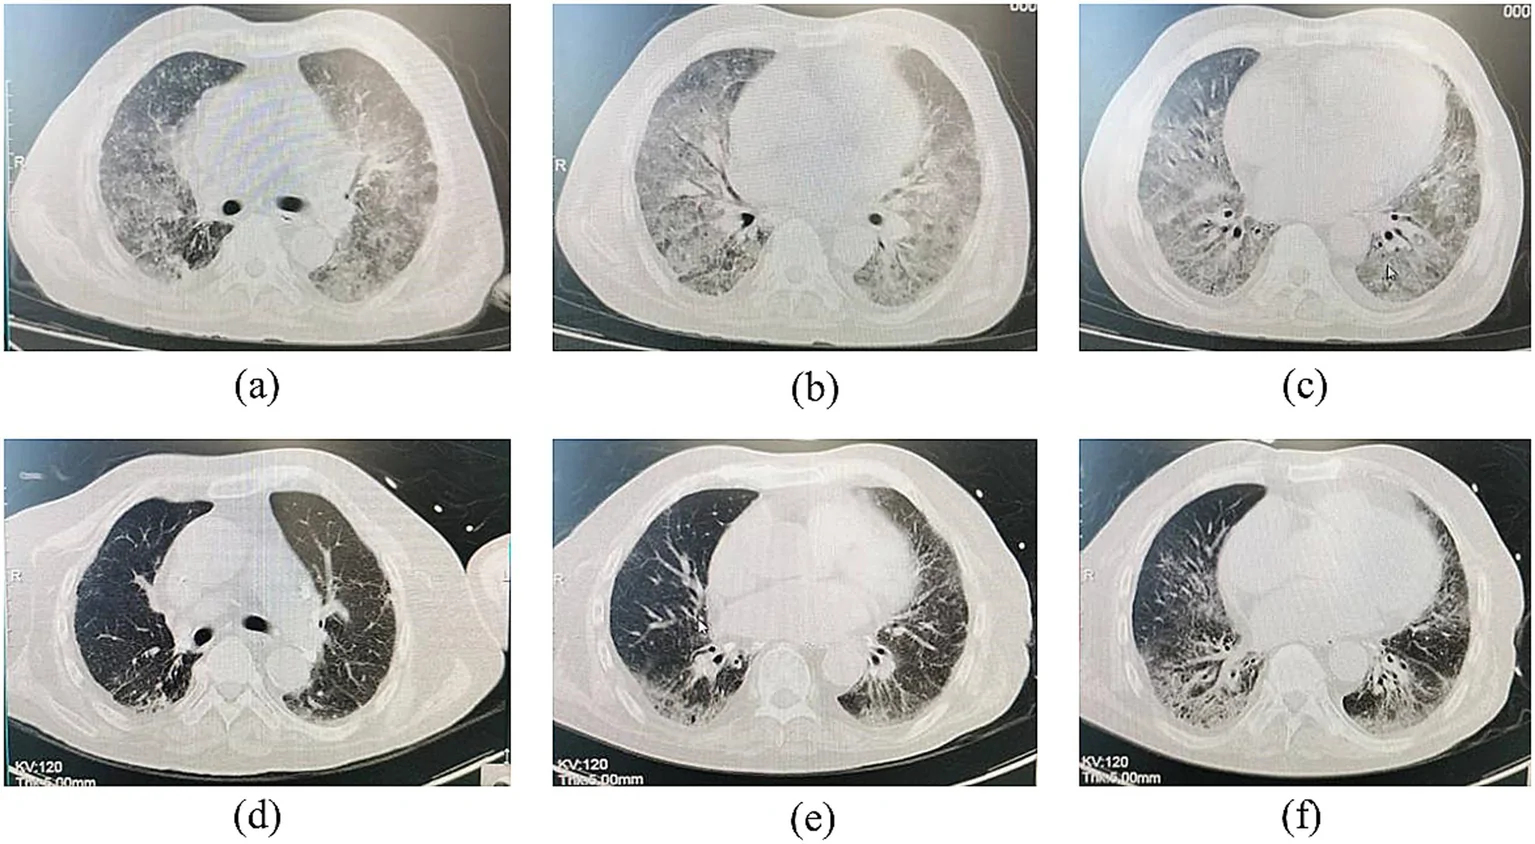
Chest CT (a–c: before treatment, diffuse exudative opacities in both lungs; d–f: after 10 days of treatment, marked resolution of exudative opacities).
